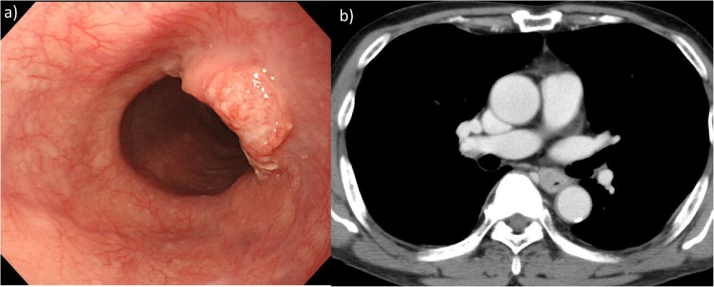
a) Endoscopic examination revealed a localized ulcerative and infiltrative tumor 27 cm from the incisors. b) The preoperative CT scan revealed no swollen lymph node.
